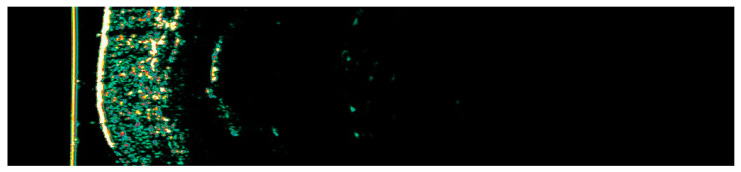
Ultrasonographic image of BCC on the cheek.
